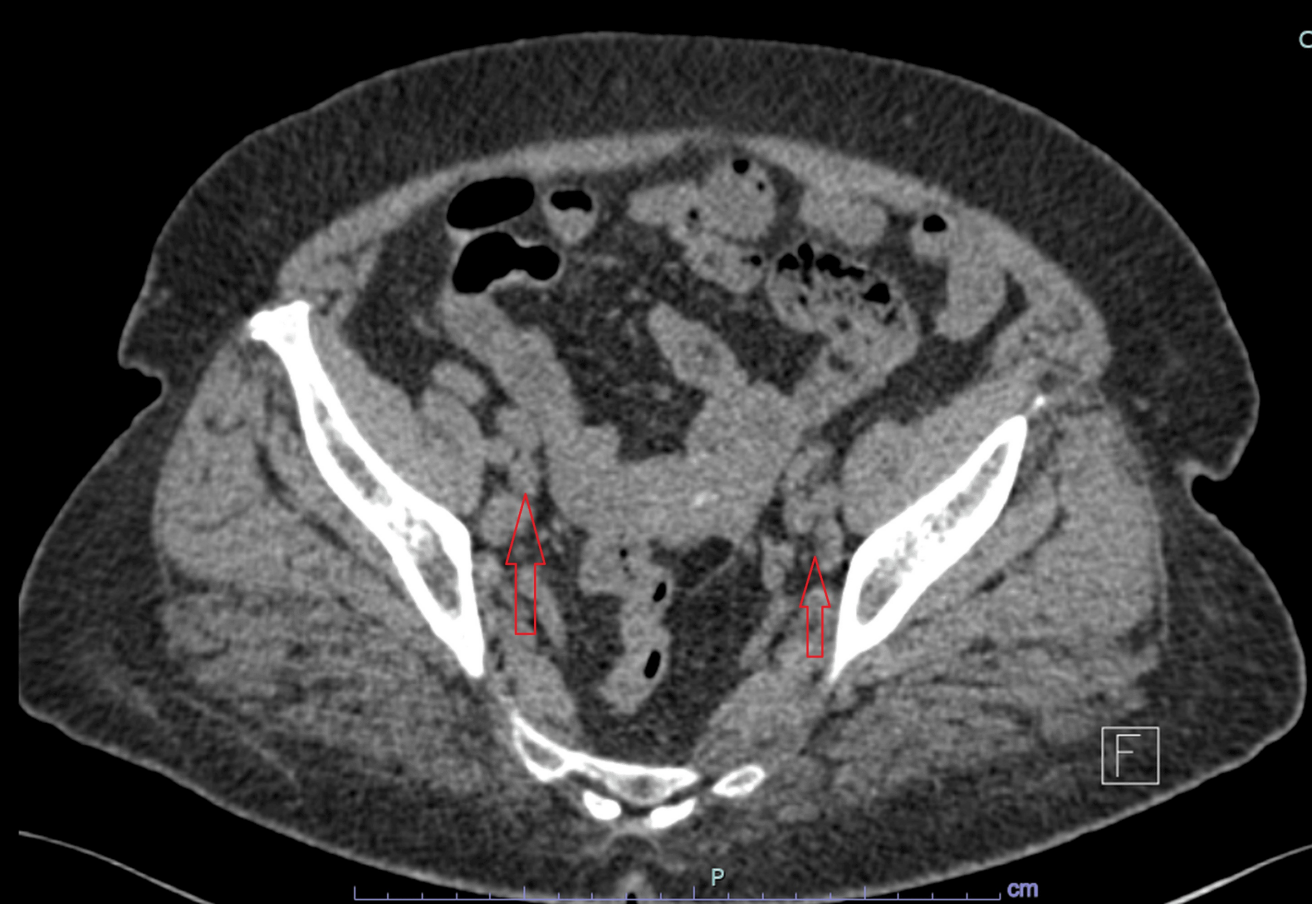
CT scan of the pelvis showing multiple enlarged lymph nodes
